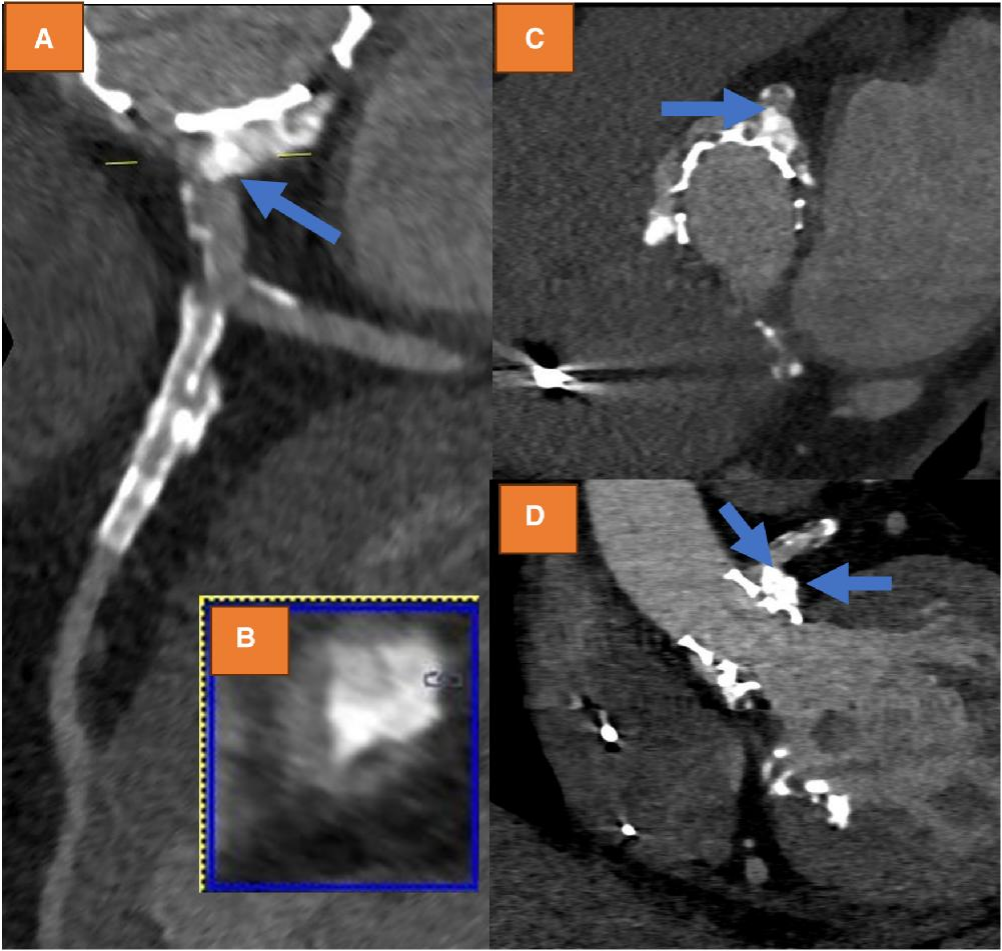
(*A*) Axial, (*B*) circumferential, (*C*) sagittal, and (*D*) coronal cardiac computed tomography angiography images of after the transcatheter aortic valve implantation procedure, demonstrating left main compression by a calcium nodule, causing high-grade left main obstruction.
